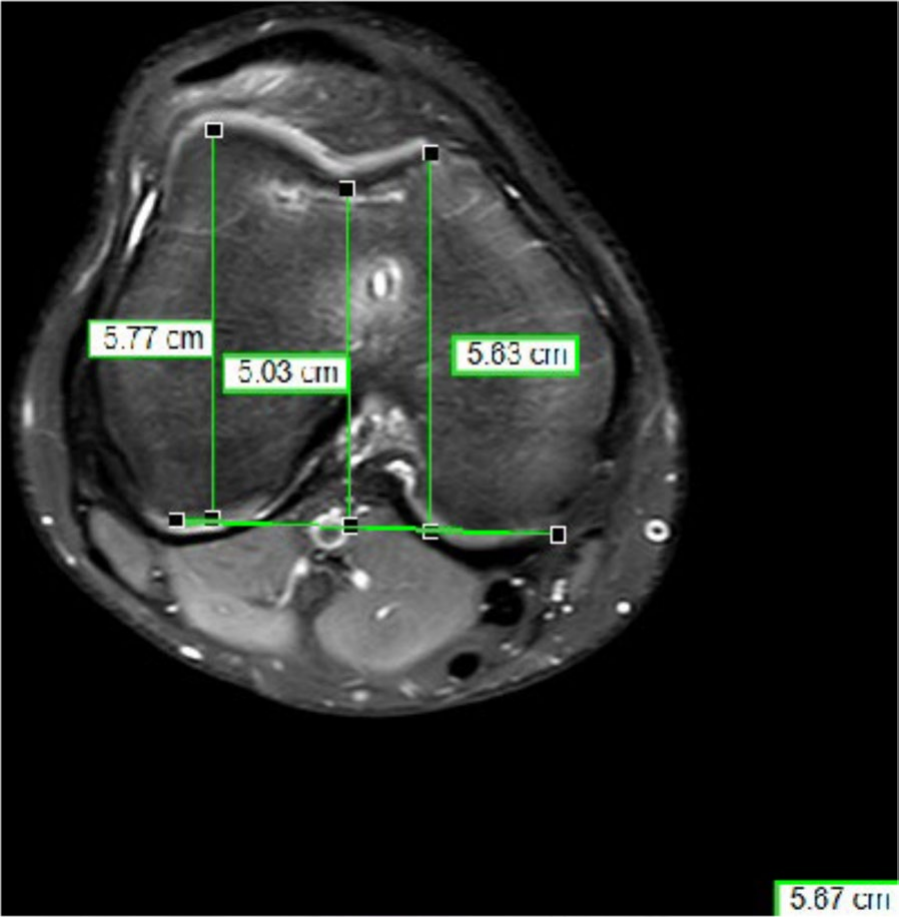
Measurement of the trochlear depth: The trochlea depth is measured in the MRI slices 3 cm above the femorotibial joint space by measuring the maximal anteroposterior distance of the medial (distance a) and lateral femoral (distance b) condyle and the minimal anteroposterior distance between the deepest point of the trochlear groove and the line paralleling the posterior outlines of the femoral condyles (distance c). Trochlear depth was calculated by the formula ([a + b]/2) − c
